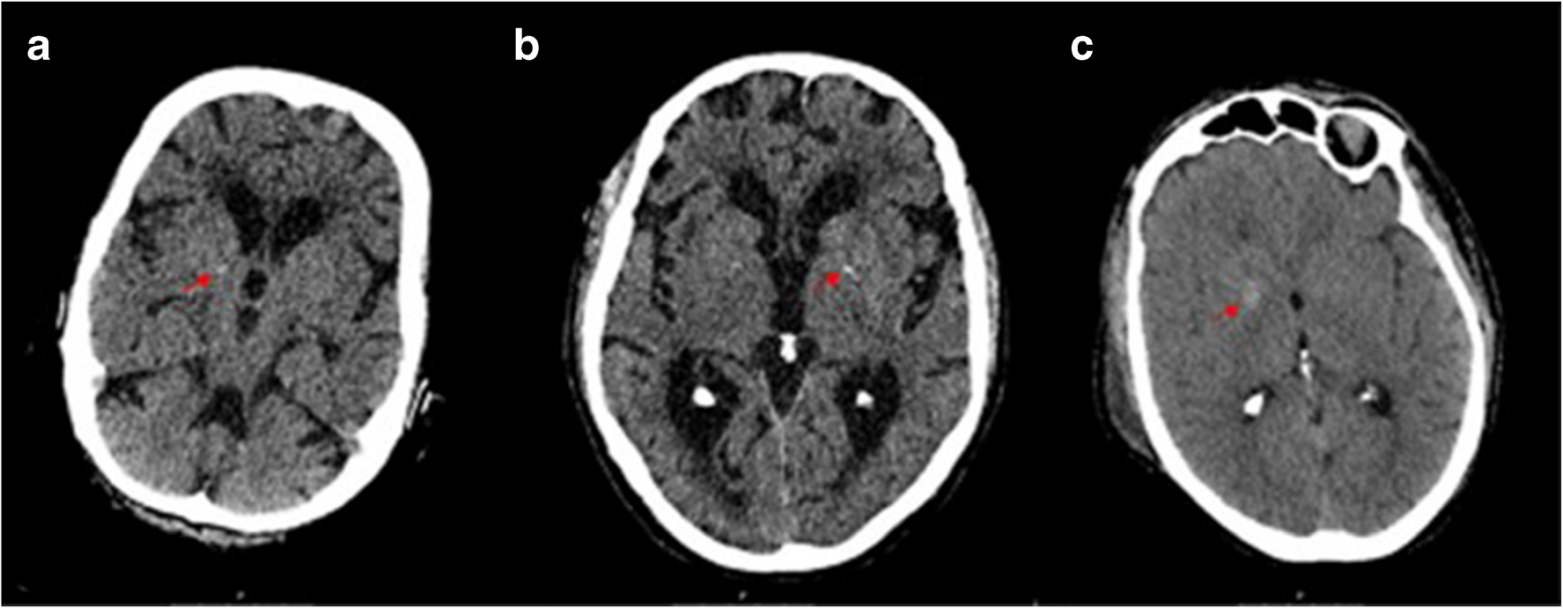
a CT scans with mild calcification in the right basal ganglia. b Moderate calcification in the left basal ganglia. c Severe calcification in the right basal ganglia
